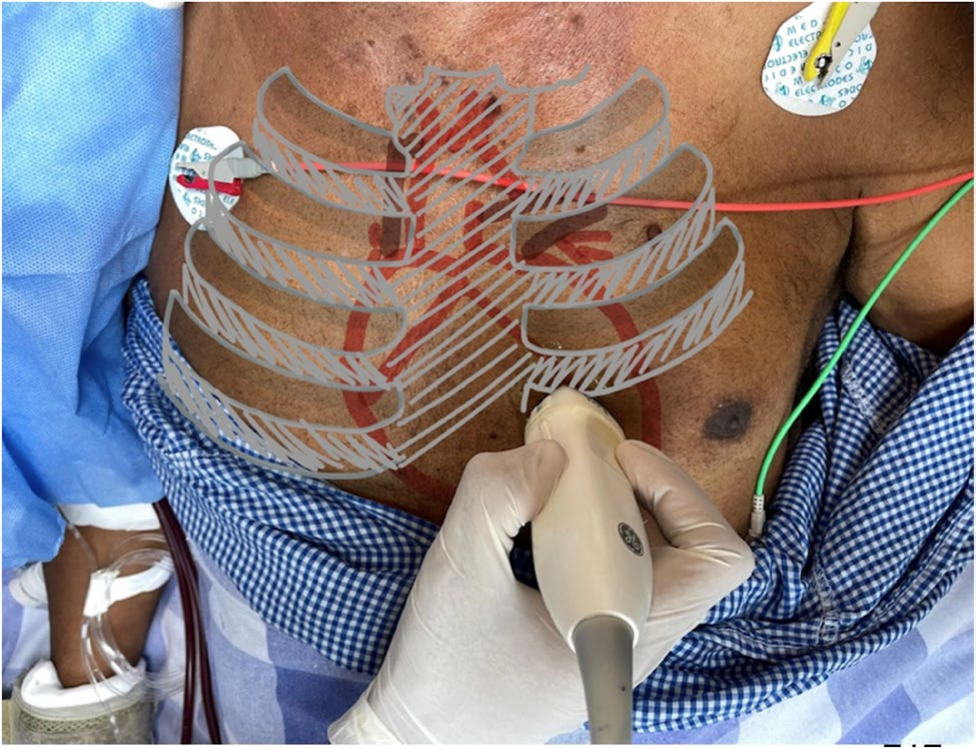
Probe placement for parasternal long axis view. Depicts prove placement in the parasternal position to obtain the PLAX view for EPSS Measurement. PLAX = Parasternal Long Axis
